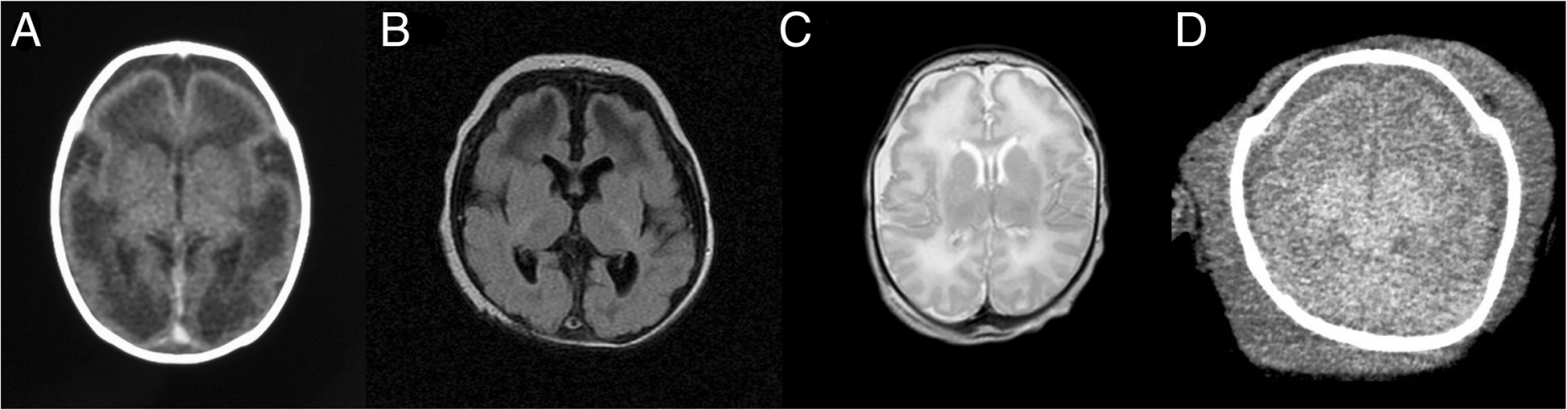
Brain images of the patients. a Non-contrast enhanced axial carnial CT scan of patient II at 2 days of age showed pachygyria involving bilateral cerebral hemispheres. b Axial sections of MRI of patient III-1 at 9 weeks of age showed gyral abnormalities, frontal pachygyria, and deficient myelination. c MRI of patient III-2 at 6 days of age showed pachygyria and hypomyelination with T2 high signal intensity of white matter in bilateral frontal and temporal lobes. d Non-contrast enhanced axial carnial CT scan of patient V at 1 month of age showed pachygyria involving bilateral cerebral hemispheres
